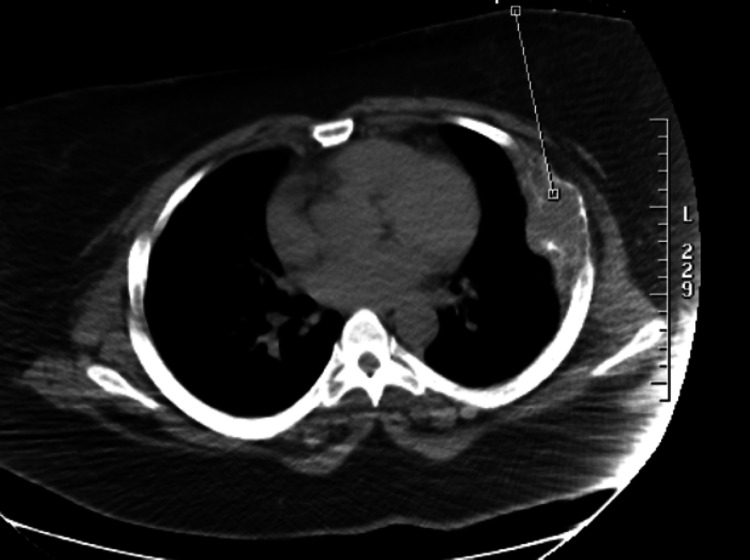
CT-guided left fifth rib biopsy
